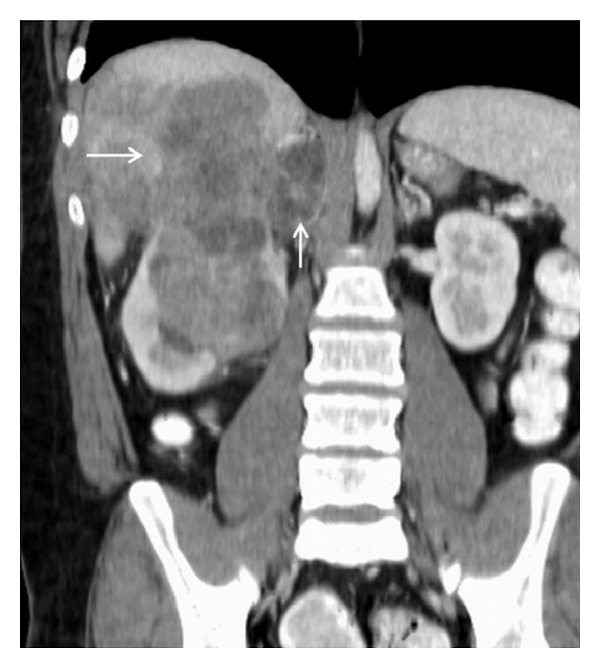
Coronal reconstructed CT image shows large lobulated mass of heterogenous attenuation invading the right liver (horizontal arrow). The right adrenal gland is expanded and completely replaced by the tumor (vertical arrow).
